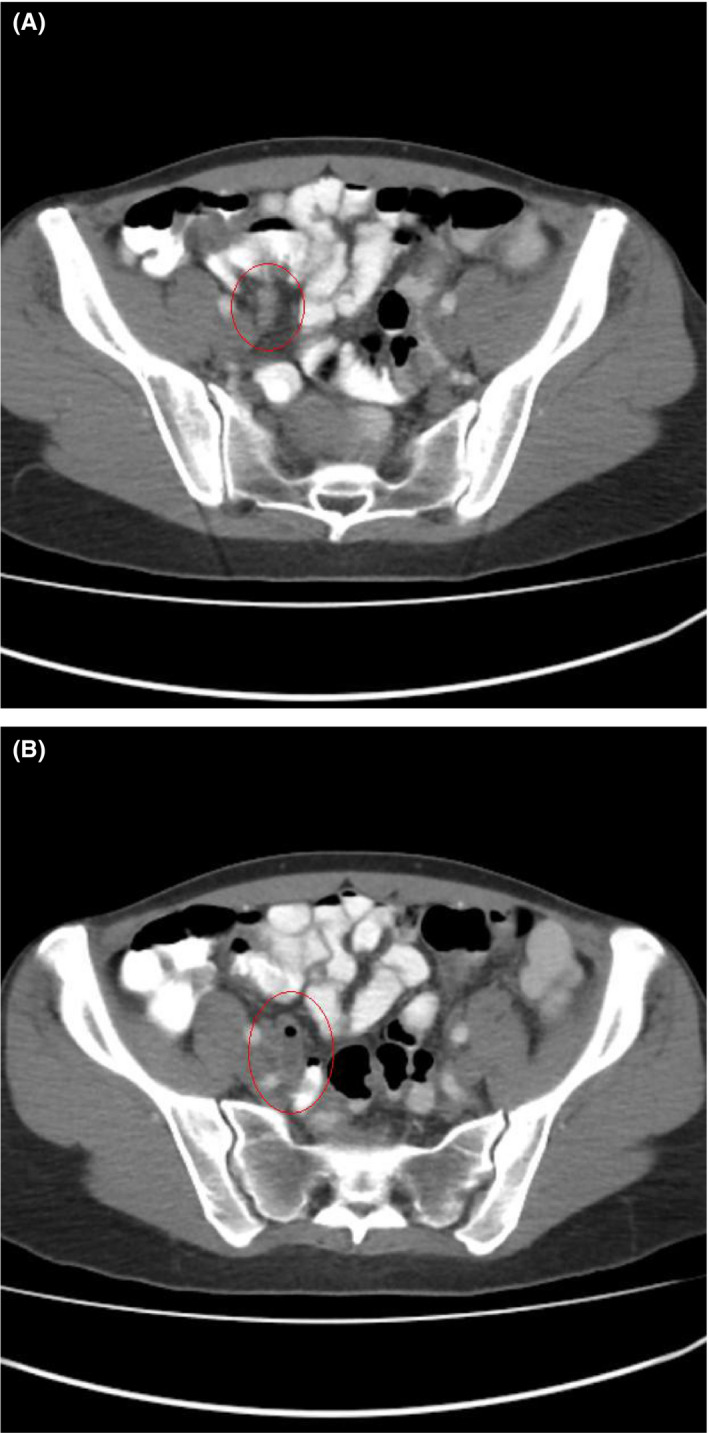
CT revealed 9‐mm wall thickening of the appendiceal base and body (Figure 1A) and 13‐mm dilatation of appendiceal tip with fluid accumulation (Figure 1B)
